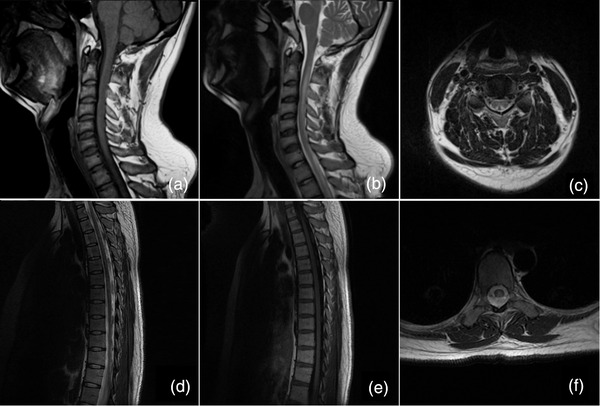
Conventional magnetic resonance imaging of the spinal cord. A 24‐year‐old man presented with impairment but was able to walk unsupported and numbness in all extremities. Sagittal T1‐weighted imaging showed decreased intramedullary signal intensity along the posterior column of the spinal cord extending from C1 to C5 (a) with corresponding hyperintensities on T2‐weighted imaging (b). Axial T2‐weighted imaging at the C4 level showed an inverted V‐shaped hyperintensity (c). A 23‐year‐old woman in a wheel‐chair presented with numbness in all extremities. Sagittal T1‐weighted imaging showed abnormal hypointensities involving the posterior columns of the spinal cord extending from T2 through T4 (d) with corresponding multiple increased intramedullary signal intensities on T2‐weighted imaging (e). An inverted V‐shaped hyperintensity was seen on axial T2‐weighted imaging at the T4 level within the dorsal thoracal spinal cord (f)
